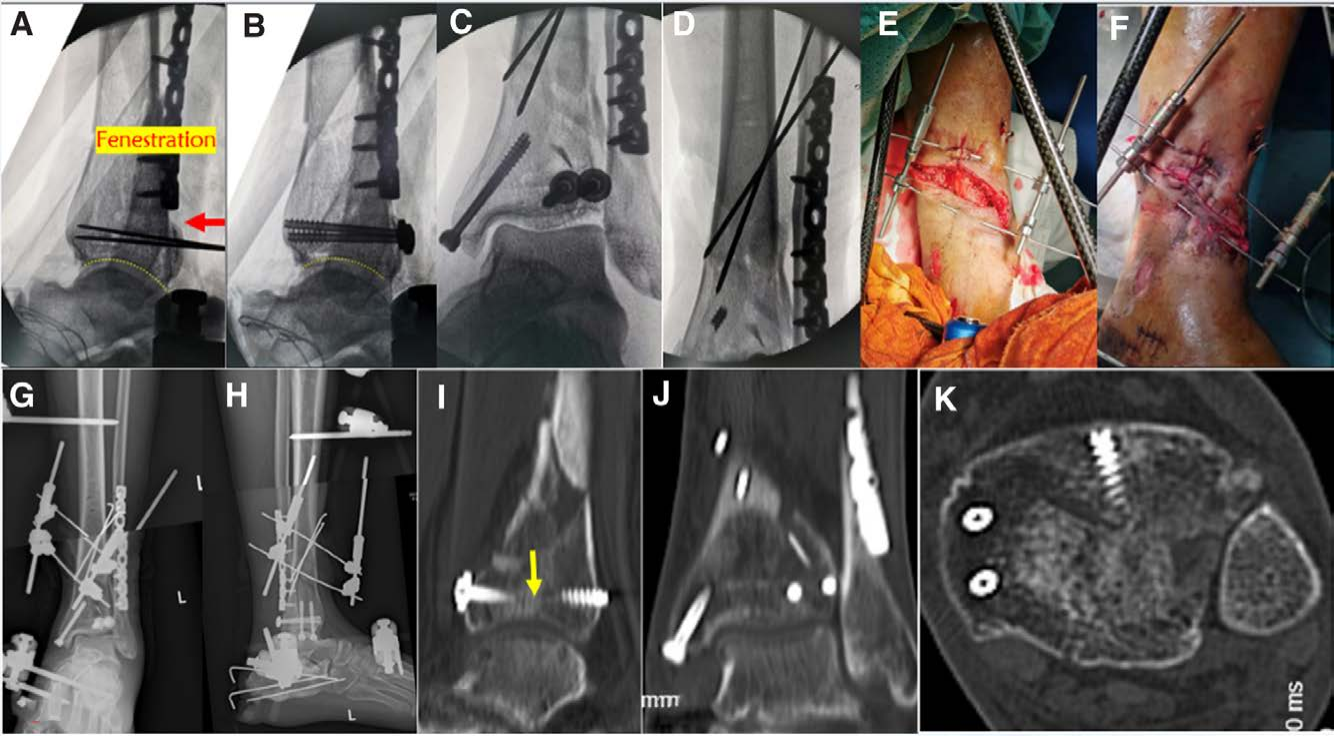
The distal articular surface of the tibia was well reduced after posterior tibial fenestration indirect reduction and compression (A and B). External fixation combined with Kirschner pin for fixation of distal tibia fracture (C and D). Closure of medial tibial wounds using skin retractors (E and F). The postoperative X-ray revealed a well-aligned fracture end force line and reduced articular surface (G and H). CT scan at 6 weeks postoperative showed that the articular surfaces were well repositioned and aligned (I–K). CT = computed tomography.
